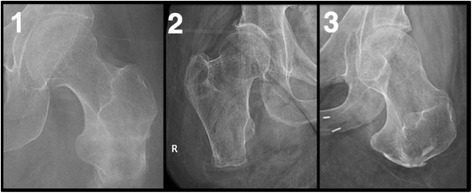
Radiographs of the residual bones for Case 1, 2 and 3
